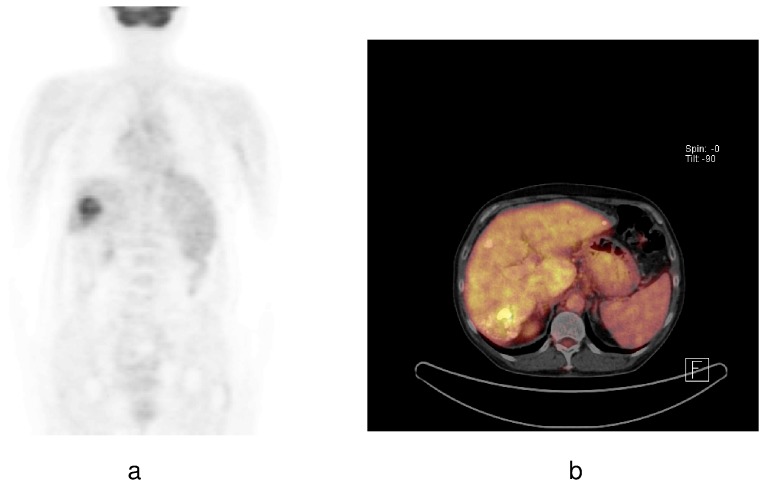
HCC with increased ^18^F-FDG uptake on *a* pretransplant PET scan and *b* PET-CT scan were classified as PET +.
